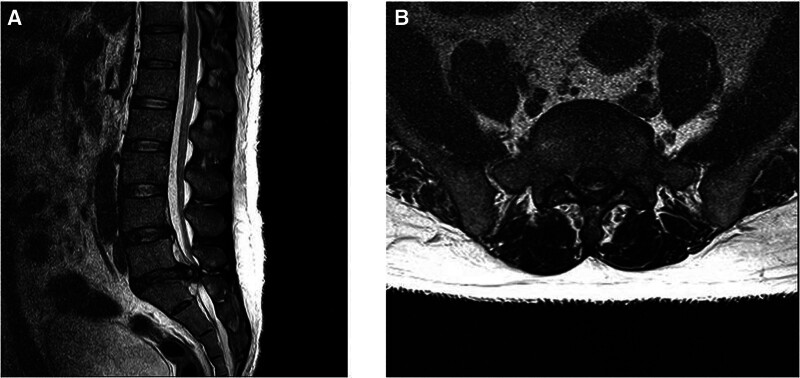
(January 07, 2022) L-spine magnetic resonance imaging: preoperatively. (A) T2 sagittal view; (B) T2 axial view.
